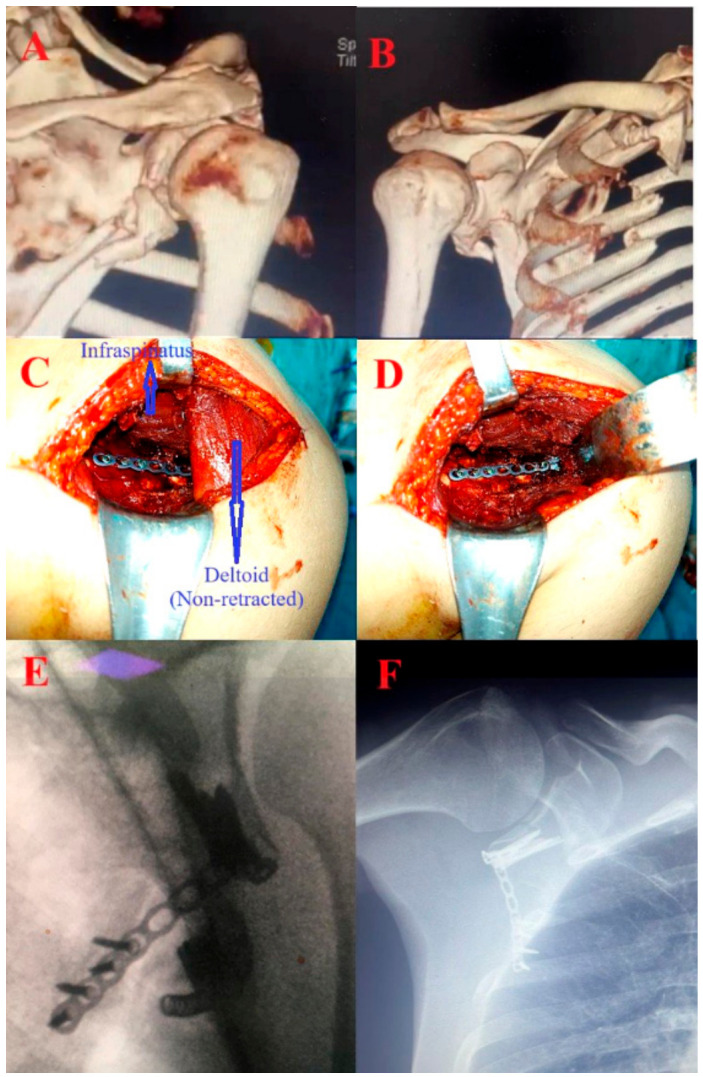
Representative intraoperative and radiographic images. (A) Preoperative three-dimensional computed tomography, posterior view. (B) Preoperative three-dimensional computed tomography, anterior view. (C) Intraoperative view after open reduction and internal fixation (ORIF) with the deltoid muscle in its native, non-retracted position. The infraspinatus muscle (upper arrow) and deltoid muscle (lower arrow) are indicated. The teres minor muscle lies immediately deep to the inferior retractor and is not visible in this view. (D) Intraoperative view after ORIF with the deltoid retracted superiorly by a retractor, providing wider exposure of the posterior glenoid and the underlying musculature. (E) Intraoperative fluoroscopic image confirming implant position after ORIF. (F) Postoperative anteroposterior radiograph demonstrating implant position and fracture reduction.
